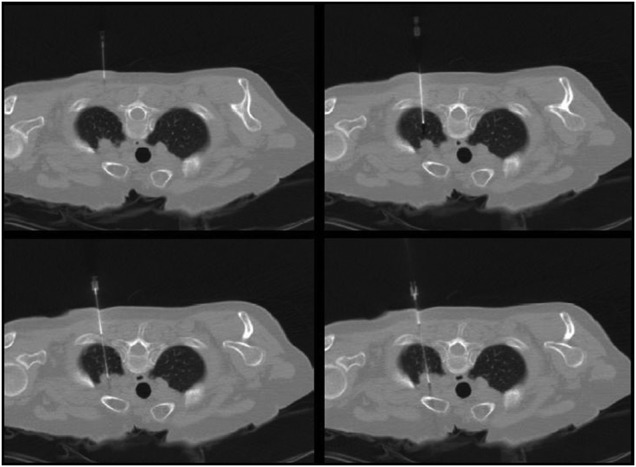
Cross-sectional image of the body with the biopsy needle being progressively inserted. Successive CT images provide visualisation of the needle location, and as such provide critical guidance to the specialist
